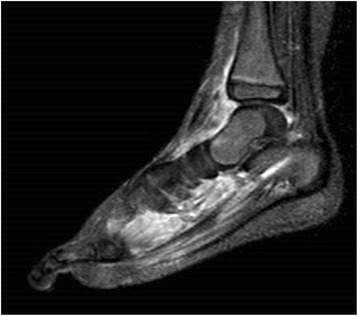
Magnetic resonance imaging of the right foot using short-tau inversion-recovery sequence revealed high signal intensity (*white*) in the soft tissue from the ankle to toe, indicating inflammatory changes
